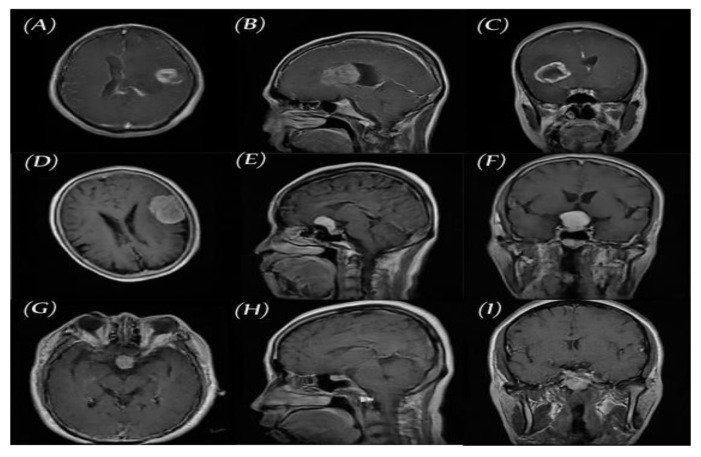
Samples of ‘realistic’ synthetic MR images, in three planes, produced by PGGAN: (A–C) glioma (D–F) meningioma, and (G–I) pituitary tumor.
